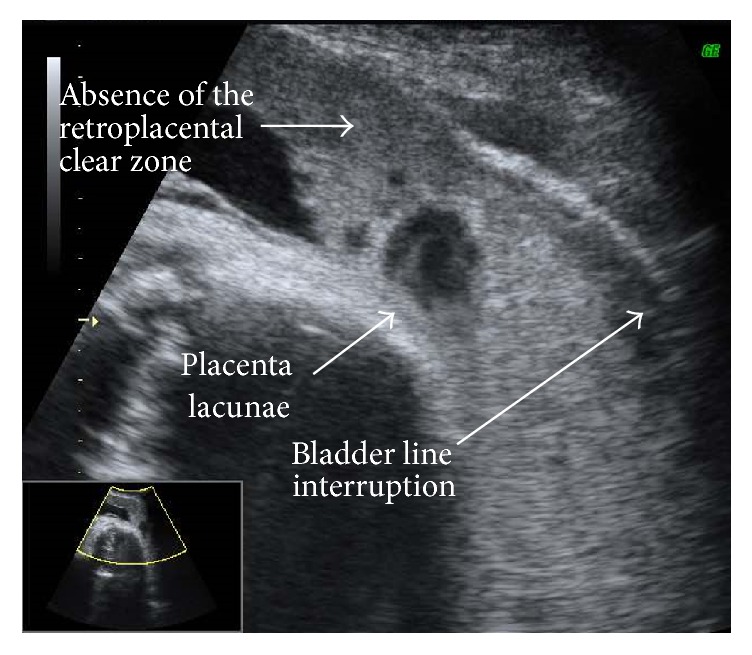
Representative 2D gray scale ultrasound scan for morbidly adherent placenta. Ultrasound scan shows bladder line interruption, absence of the retroplacental clear zone, and placenta lacunae in morbid adherent placenta.
